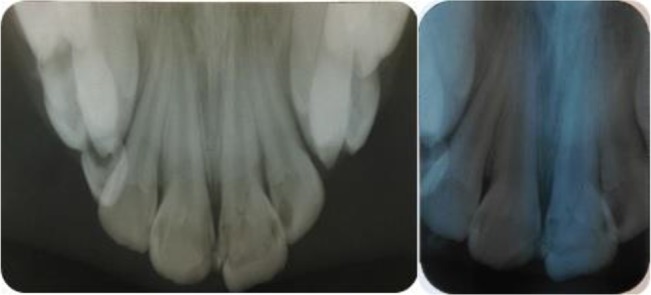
Preoperative intraoral occlusal(left) and periapical(right) radiographs demonstrating oblique complicated crown-root fracture affecting the left central incisor
